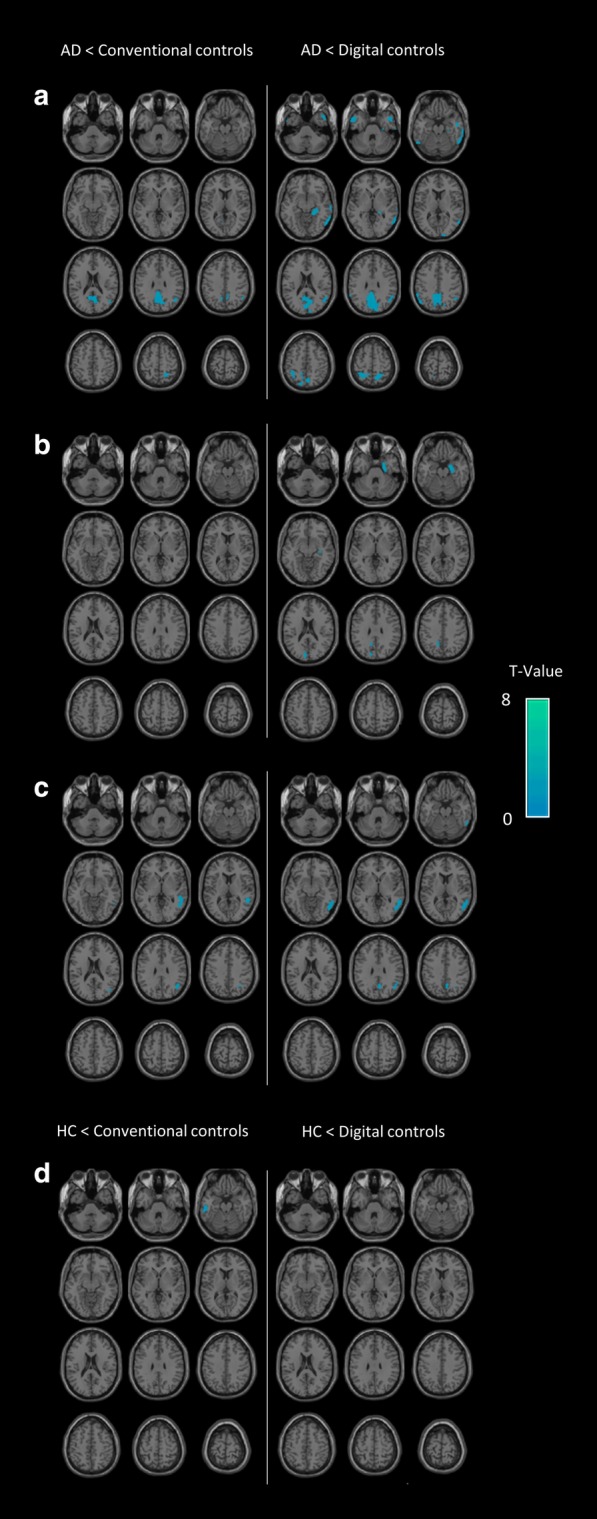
Galleria of individual examples with both semi-quantitative analyses to conventional and digital controls. Three examples of SPM-T map images from semi-quantitative analyses to conventional (left panel) and digital (right panel) controls of patients with AD (a 74-year-old woman with MMSE score of 19, b 70-year-old woman with MMSE score of 21, c 66-year-old man with MMSE score of 23). One example of SPM-T map images from semi-quantitative analyses to conventional (left panel) and digital (right panel) controls from a healthy subject (d 54-year-old woman with MMSE score of 29). SPM-T maps are projected onto two-dimensional slices of T1-weighted MRIs (from the base to the top of the skull)
